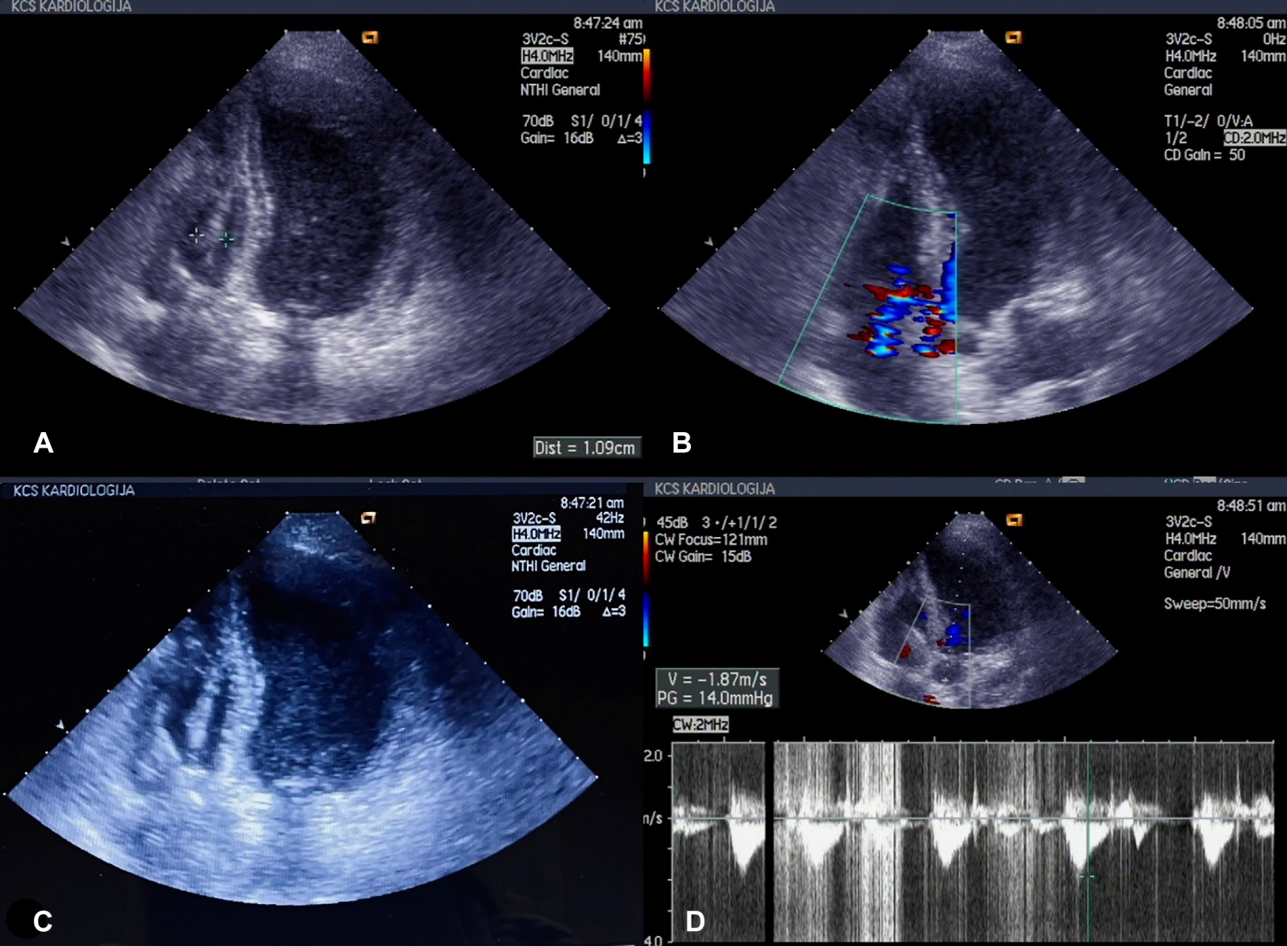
(A–D) Echonosonography showing vegetations covering the pacemaker electrode in the right ventricle.
